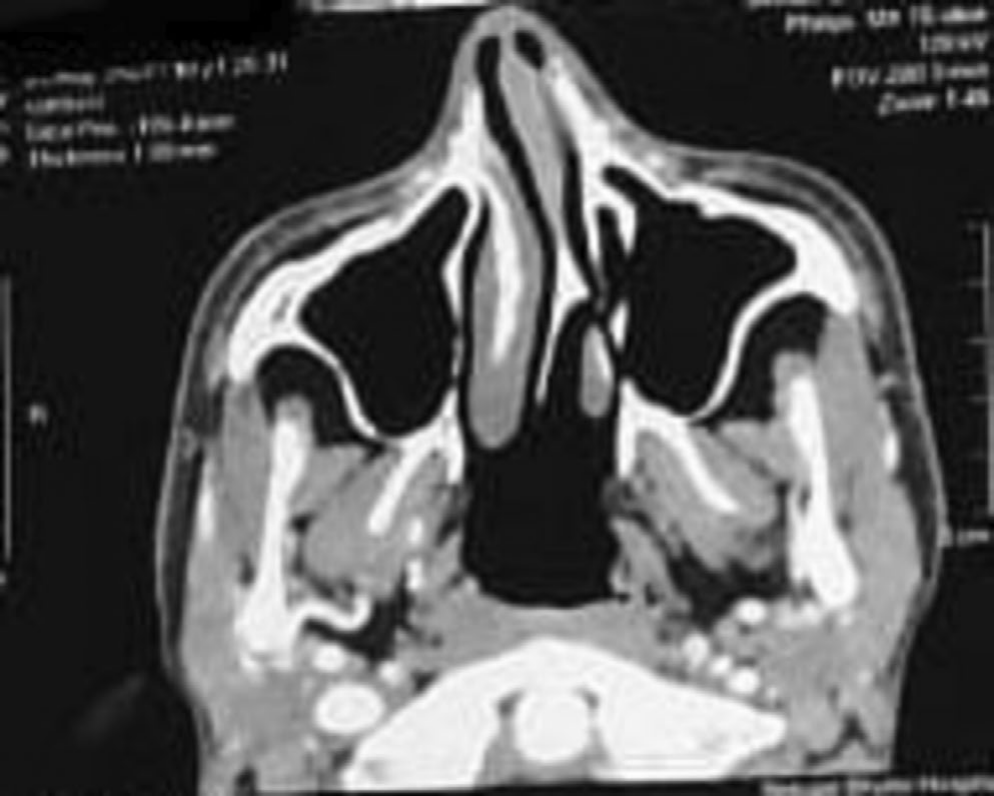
A CT scan of the nose and paranasal sinuses shows a deviated nasal septum. The CT scan further reveals compensatory inferior turbinate hypertrophy on the right side and mild bilateral ethmoidal sinusitis.
